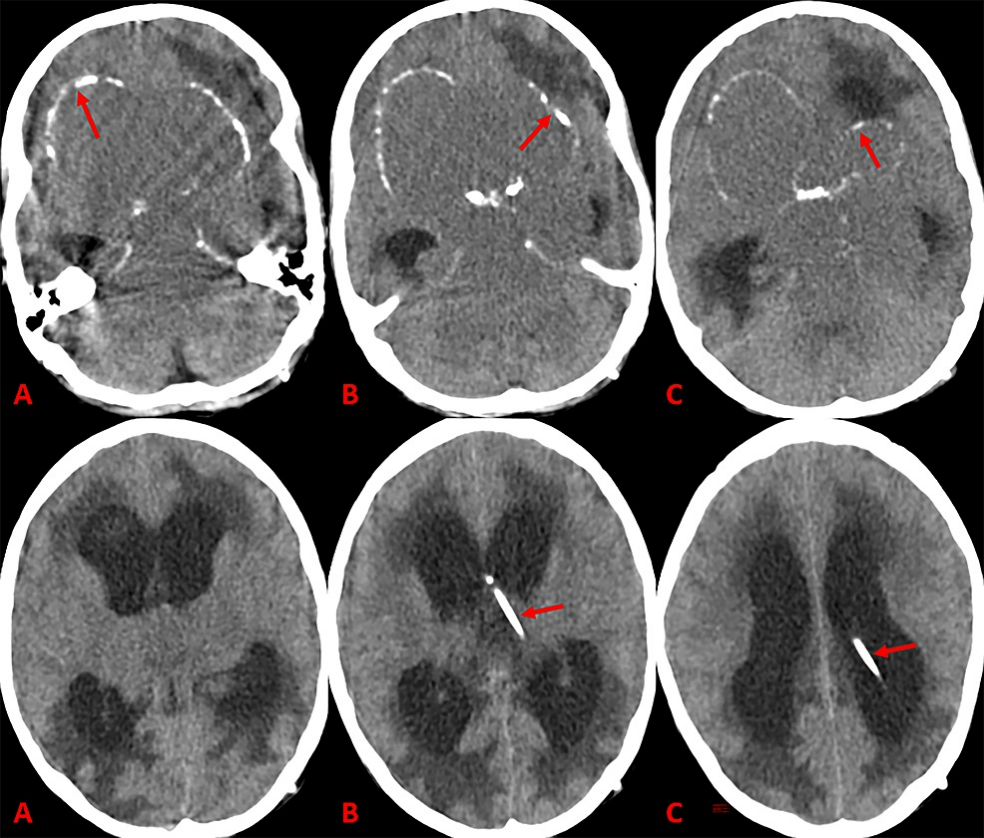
Head CT scan images reveal a massive cystic lesion with extension from the anterior to posterior fossa and upward to the lateral ventricles Cystic contents are iso-to-hypodense and peripheral punctate calcifications (arrows) are evident (A-C). Diffuse periventricular hypodensity due to interstitial edema (D, arrow) initially persisted despite shunt placement (E & F, arrows).
